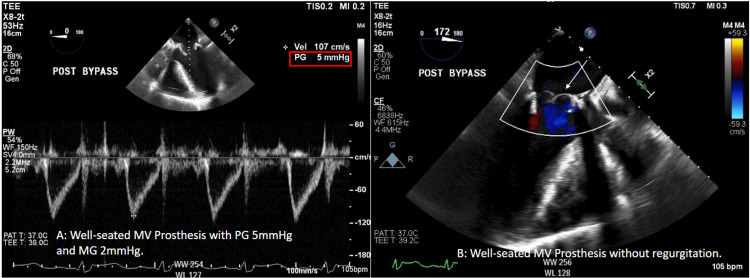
Post cardiopulmonary bypass transesophageal echocardiogram demonstrating a well seated mitral valve prosthesis with acceptable gradients (A) and no regurgitation or perivalvular leak (B). The red box and white arrow indicate relevant findings.
